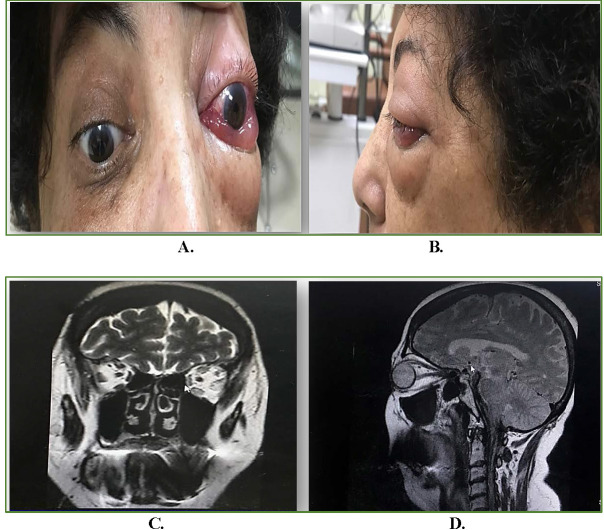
External photograph front view (A) and lateral view (B) of left eye depicting proptosis, periorbital edema, diffuse conjunctival congestion and chemosis; C. Contrast MRI (Coronal view) showing increase in bulk of all the extraocular muscles of left eye; D. MRI (Sagittal view) showing increase in bulk of all the extraocular muscles of left eye with tendon sparing
